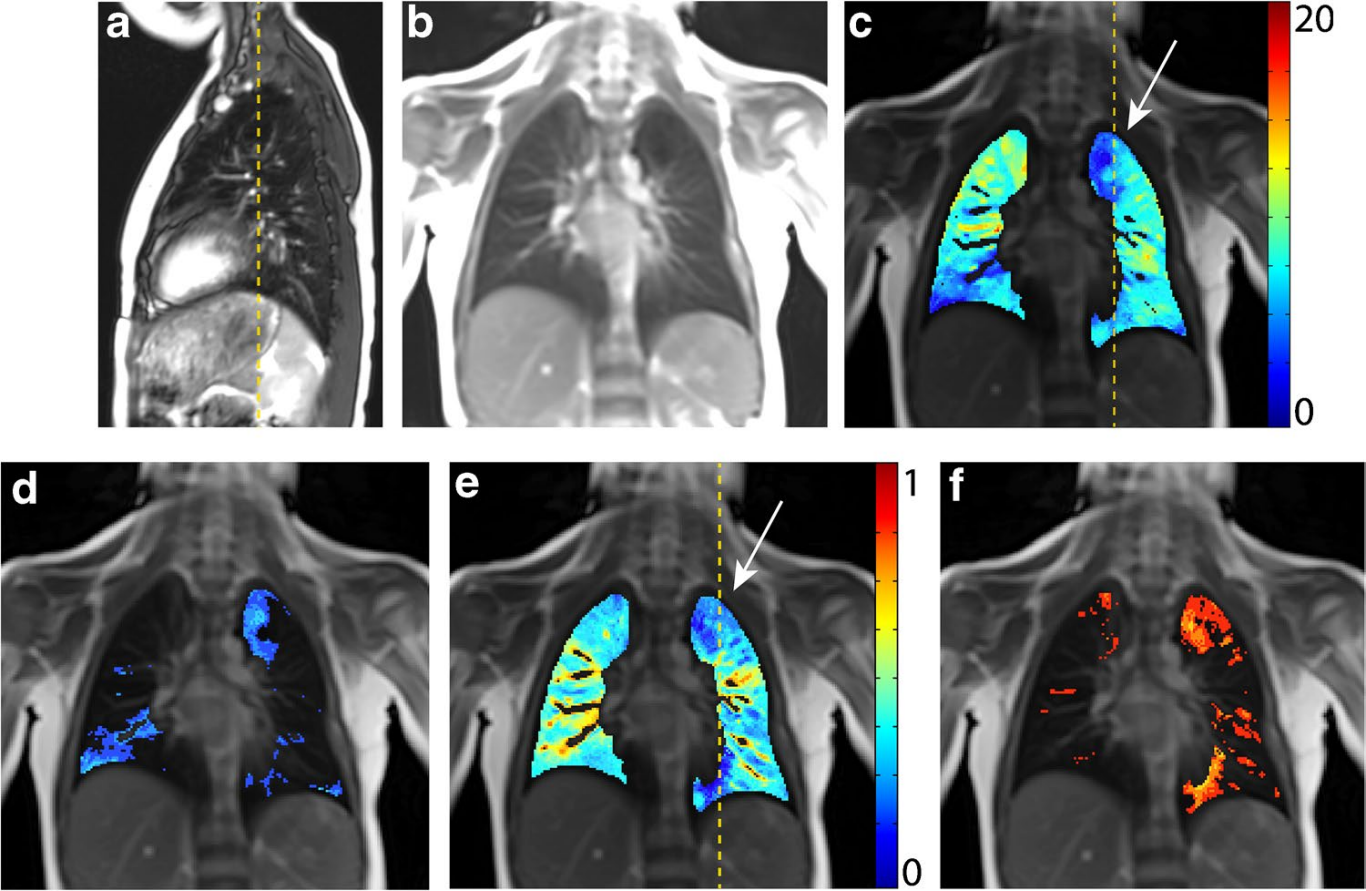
A 6-year-old girl following atypical stapler-resection of Seg I-III of the left lung for CPAM type 1 with a normal lung clearance index of 6.6 turnovers. A sagittal T2-weighted image (a) (repetition time [TR]/echo time [TE] 3.3/1.3 ms) and (b) coronal T1/2-weighted image (TR/TE 1.5/07 ms) show lung structures in the area of the former left lung segments I-III. No further abnormalities were visible. c-f Coronal functional magnetic resonance imaging maps: (c) fractional ventilation, (d) fractional ventilation defect percentage (brighter blue areas represent reduced function), (e) relative perfusion and (f) relative perfusion defect maps (brighter orange areas represent reduced function) show a fractional ventilation defect percentage (13.6%) and a perfusion defect percentage (14.5%) of the whole lung within the norm. However, in the left upper lobe, reduced fractional ventilation (c) and relative perfusion (e) are visible (*white arrow*). *Yellow dashed lines* indicate plane levels. *CPAM* congenital pulmonary airway malformation
